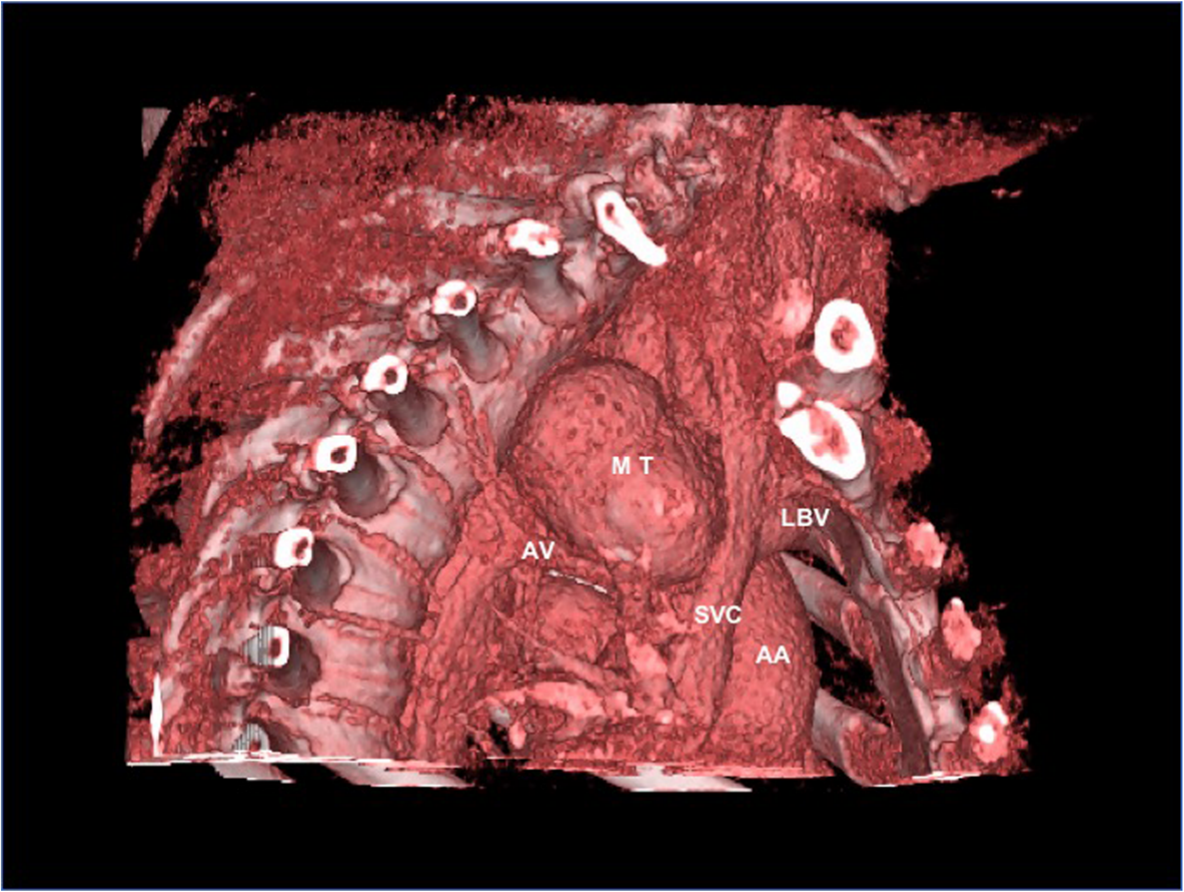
Tridimensional contrast Enhanced-CT reconstruction (AV: azygos vein; MT: mediastinal mass; SVC: superior vena cava; AA: ascending aorta; LBV: left brachiocephalic vein)
